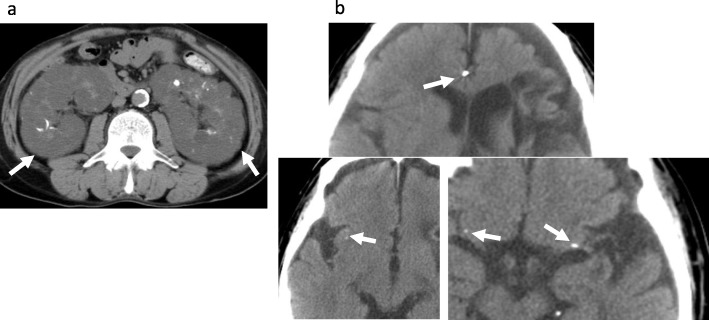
Radiologic findings in the proband’s brother. a Unenhanced computed tomography scan shows multiple kidney cysts (*arrow*) and b brain nodule (*arrow*)
